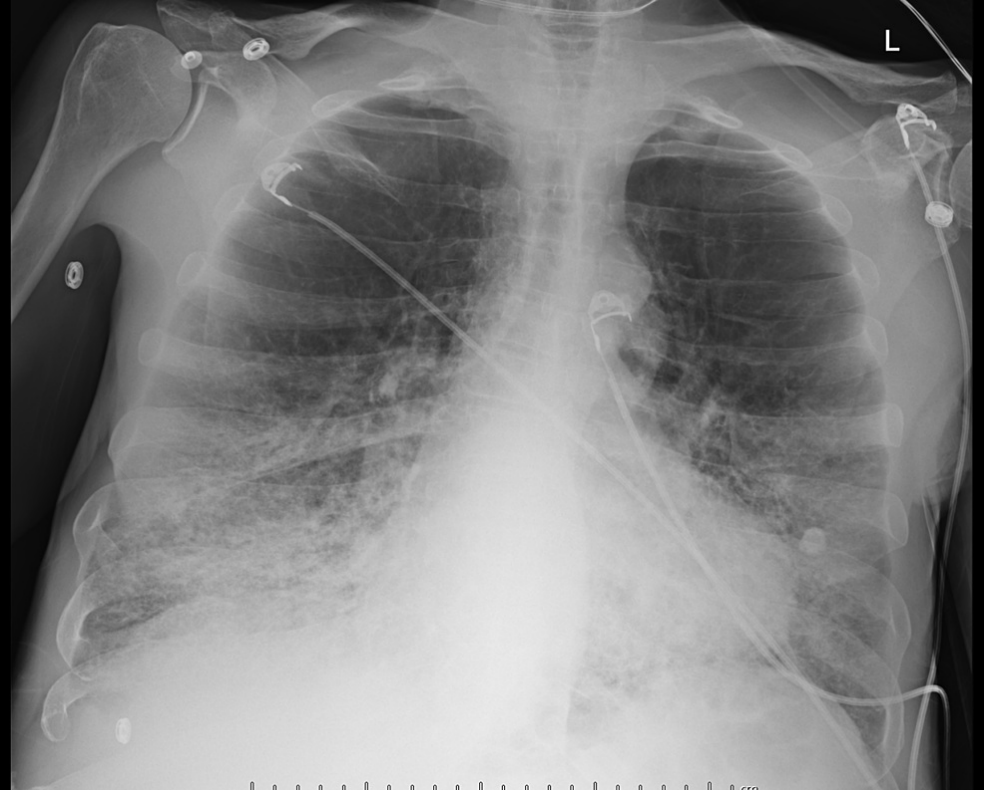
Radiography showing bilateral airspace disease typical of COVID-19
